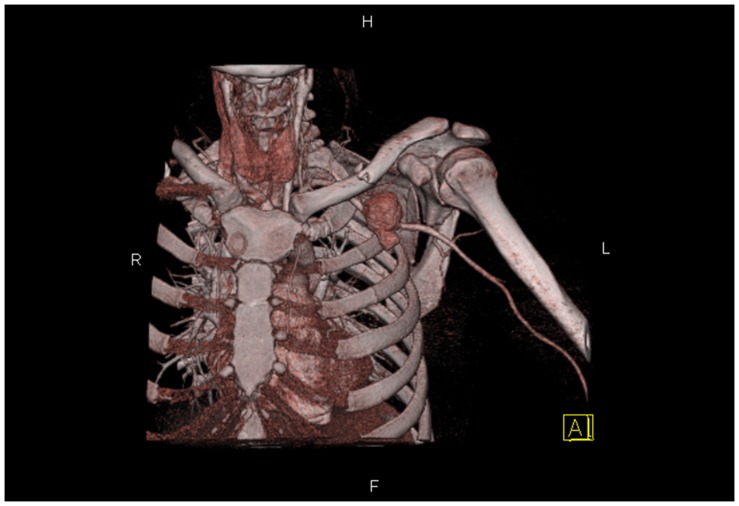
The result of CTA for patient no. 6. Computed Tomographic Angiography (CTA) of patient no. 6 showed the rupture was in the anterior wall of axillary artery.
